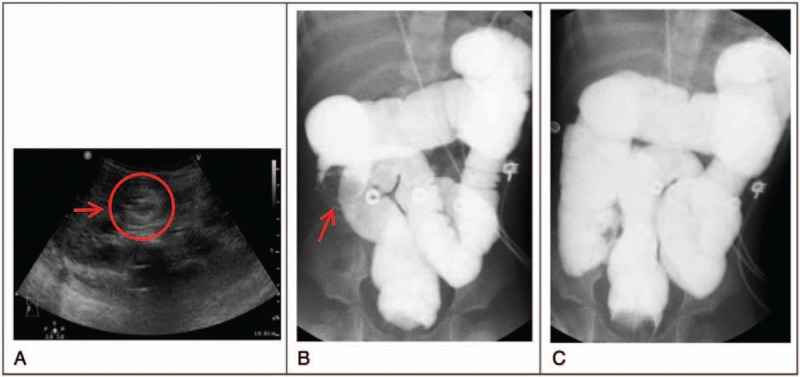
Twin B. A, Abdominal sonography displaying the target lesion (red arrow). B and C, Lower gastrointestinal series revealed ileocolic intussusception (red arrow) and successful reduction.
